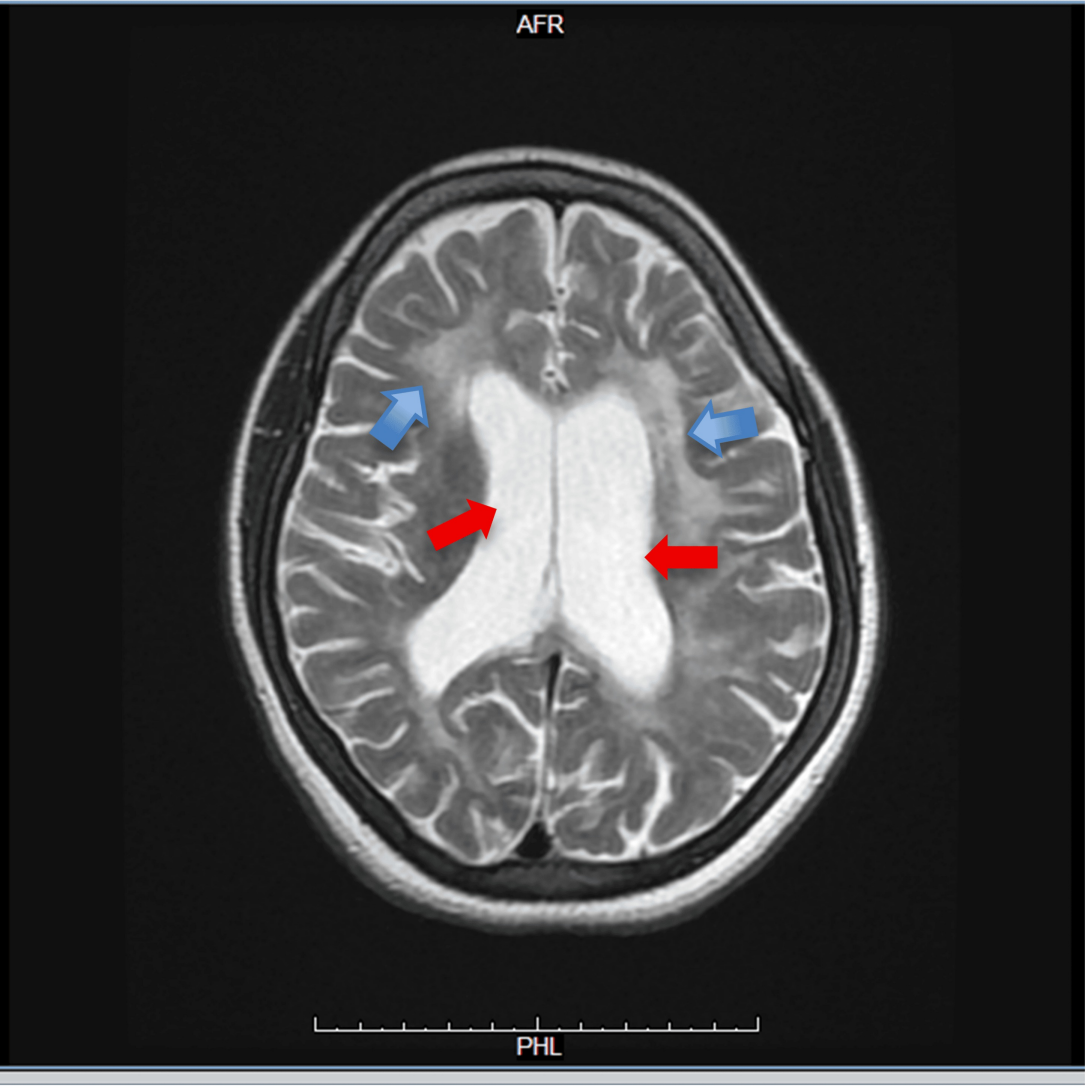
Axial T2 FSE-weighted MRI of the brain Axial T2-weighted MRI shows bilateral periventricular and deep white matter patchy hyperintensities (blue arrows) with enlarged ventricles (red arrows) and diffuse brain volume loss disproportionate to age. No restricted diffusion, hemorrhage, hydrocephalus, or mass effect is present. The findings are stable compared to previous MRI studies and are most consistent with chronic white matter involvement in neuropsychiatric SLE MRI: magnetic resonance imaging; FSE: fast spin echo; SLE: systemic lupus erythematosus
